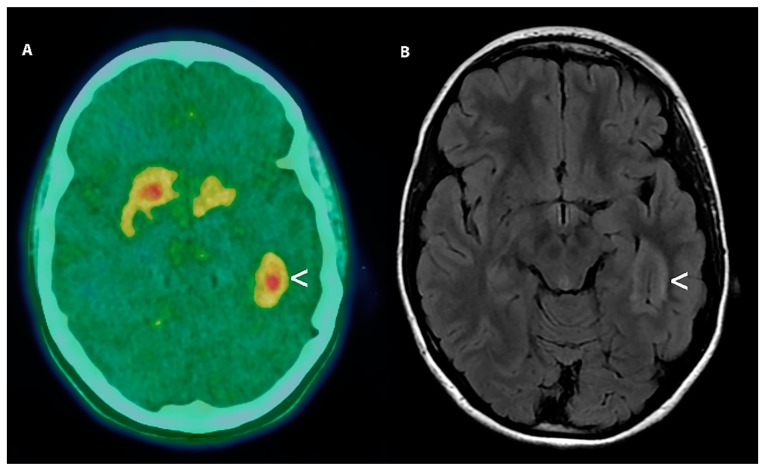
[^18^F] FDOPA PET (A) performed on September 2013 of a 16 years old male patient surgically treated for a Pilocytic Astrocytoma showing no area of focal increased uptake of the radiopharmaceutical (Standardized uptake value was equal to 0.9 vs. 0.8 of the surrounding brain tissue) (A,<). In (B) we report the axial CT scan showing the extent of surgery. Routine follows up examinations in February 2019 was negative for cancer recurrence.
